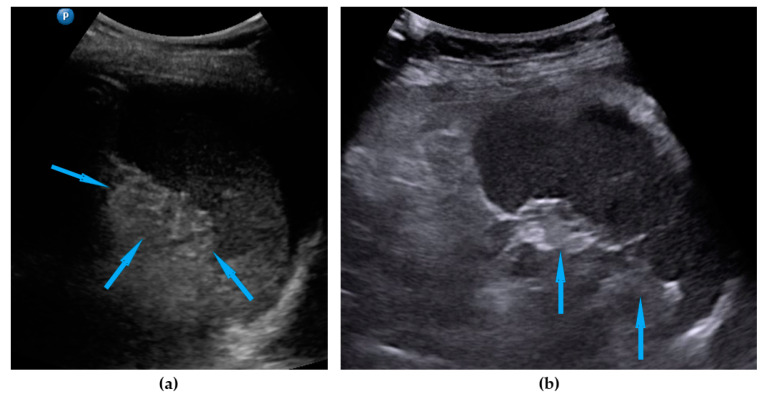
Ultrasound images of spleen hilum involvement: (a) diffuse infiltration; (b) focal lesions. Blue arrows identify lesions.
